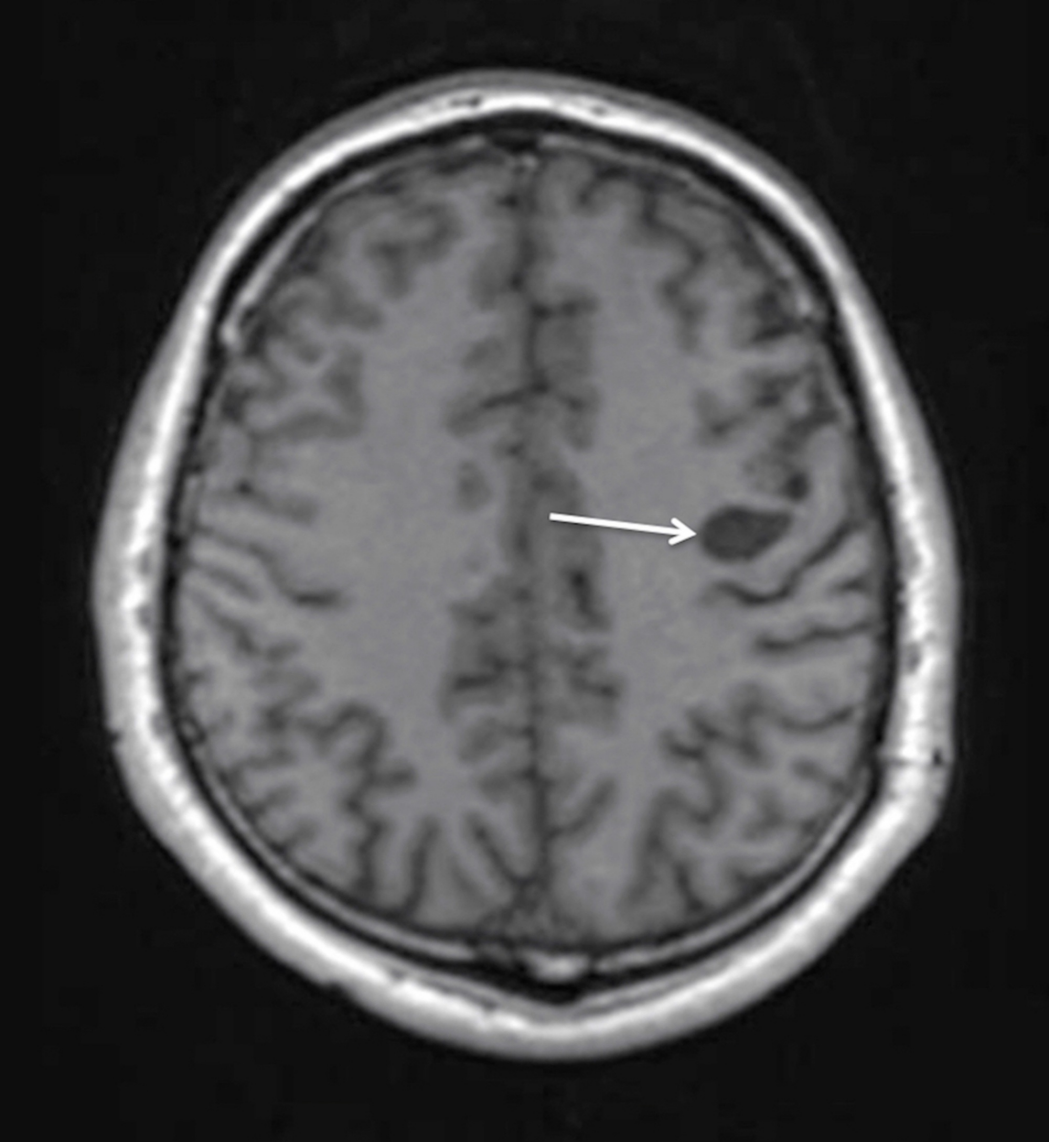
Postoperative MRI with contrast enhancement at two-year follow-up demonstrating the residual cyst (arrow) without contrast accumulation, further decrease in size, and no mass effect on the T1WI axial view. MRI: Magnetic resonance imaging; T1WI: T1-weighted images.
